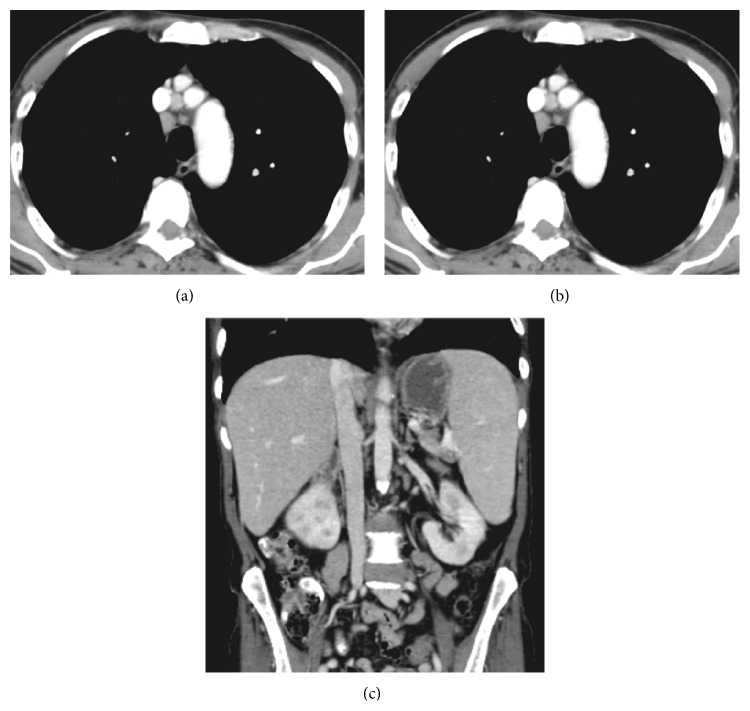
Axial postcontrast CT of the chest ((a) and (b)) shows minimally enlarged mediastinal lymph nodes; the largest one in the right pretracheal region measures about 12.3 × 11.1 mm. Coronal postcontrast CT of the abdomen (c) shows mildly enlarged spleen and liver measuring about 14.5 and 15.7 cm, respectively, without evidence of focal lesions or associated abdominal lymphadenopathy.
